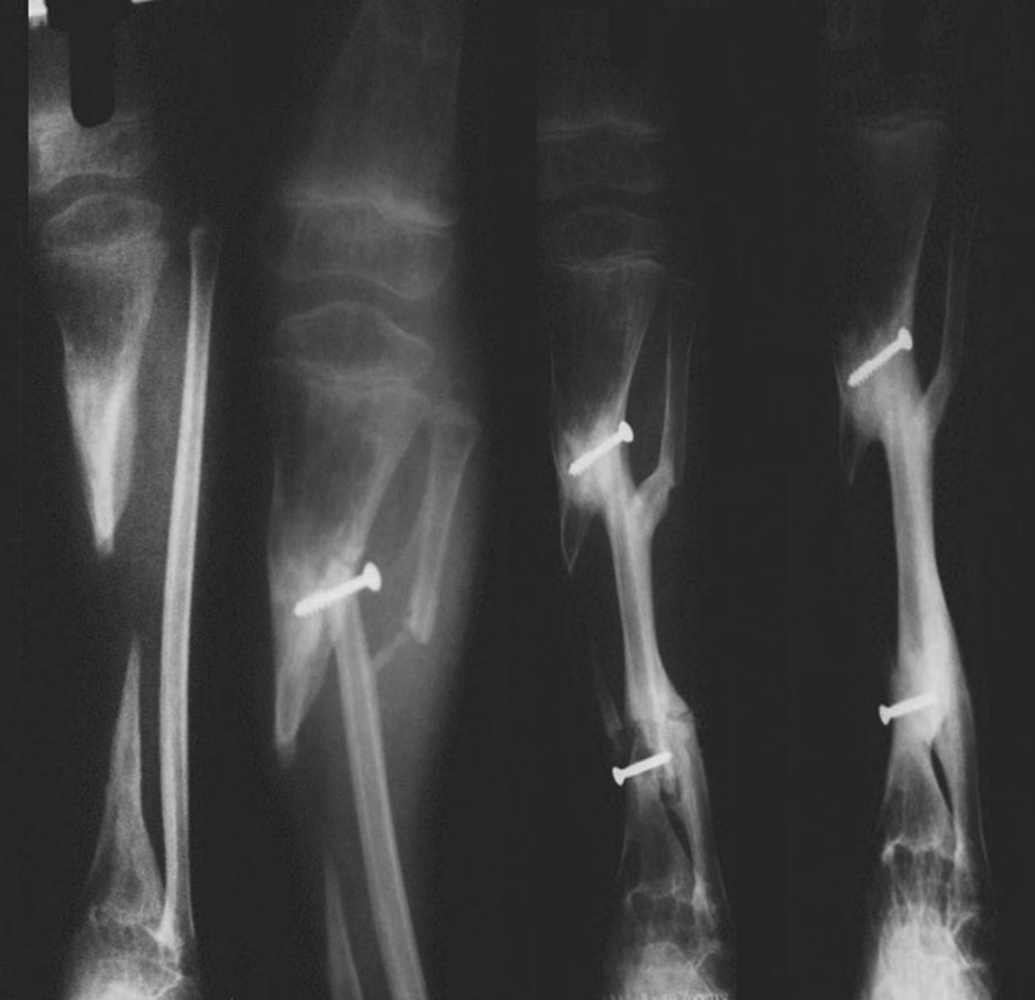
Radiographs of 10-year-old boy showing progressive consolidation of fibular graft and hypertrophy of tibialized fibula 4 years post-surgery
